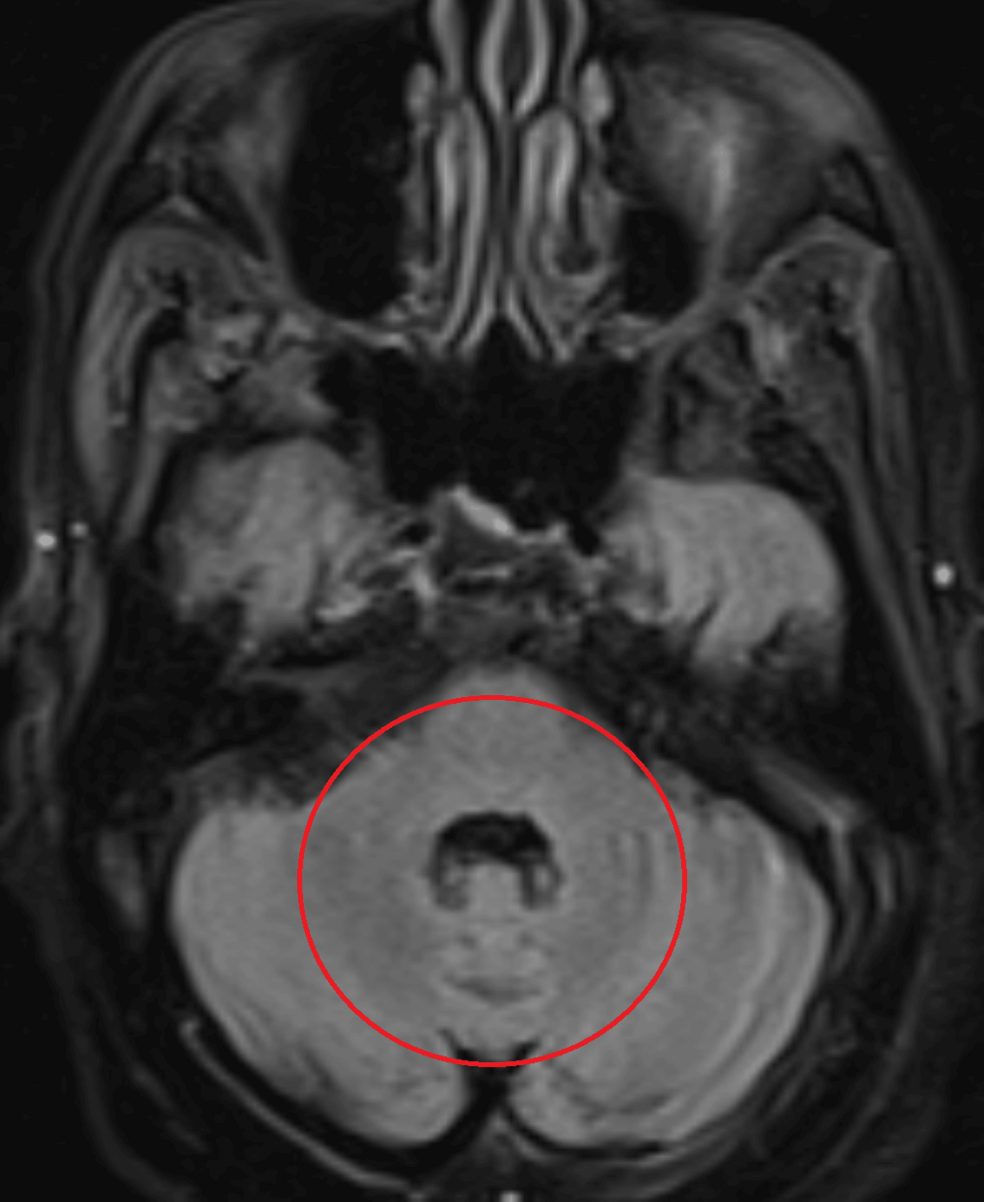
FLAIR sequence of brain MRI showing no abnormality in pons or cerebellum. The red circle shows the area of interest. FLAIR: fluid-attenuated inversion recovery
